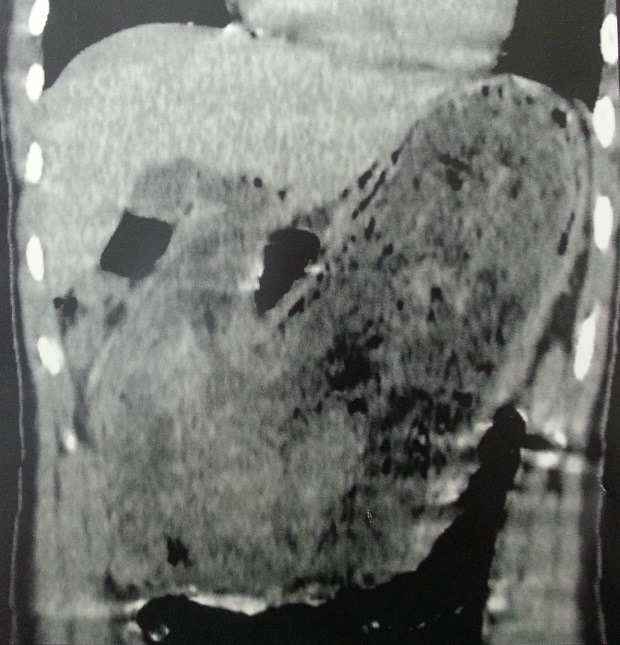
Preoperative CT scan: frontal image of the gastro-duodenal trichobezoar
